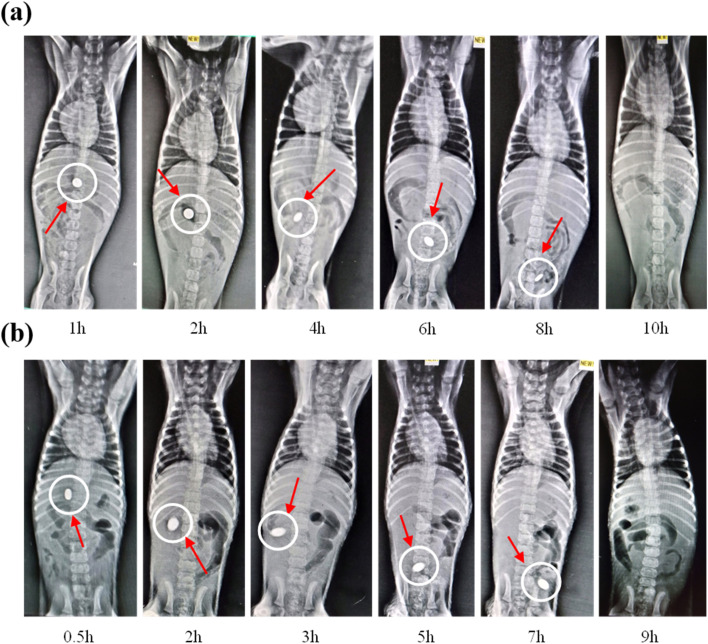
(a) *In vivo* X-ray study of XNG-based SD (O-X) at different time intervals and (b) i*n vivo* X-ray study of HPMC-based SD (O–H) at different time intervals.
